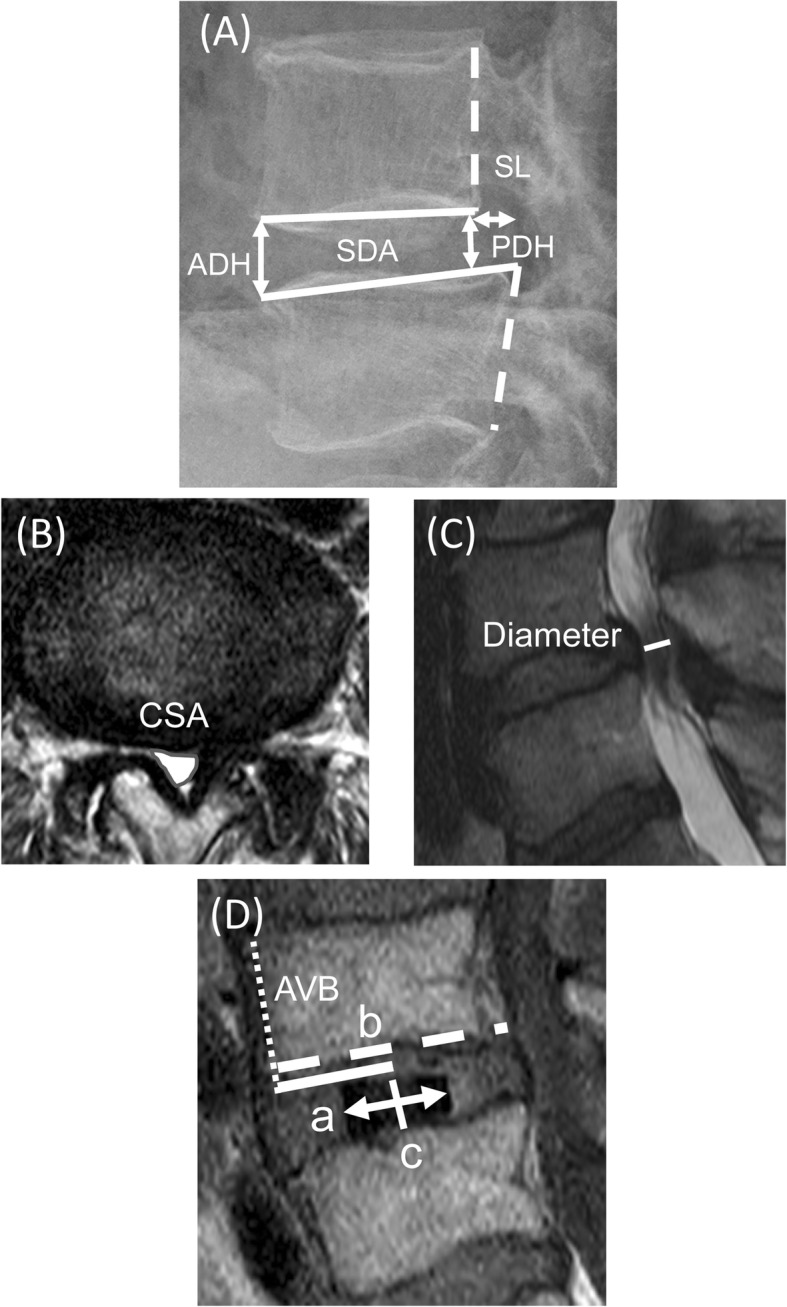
A radiographic analysis of XLIF cage positioning. (A) Plain lateral radiographs: segmental disc angle (SDA), disc height (DH), and slipping length (SL). (B) Magnetic resonance imaging (MRI)-T2 axial: cross-sectional spinal canal area (CSA). (C) MRI-T2 sagittal: diameter of the spinal canal. ADH; anterior disc height, PDH; posterior disc height. (D) The cage centre (c) is located by the midpoint between the anterior and posterior radiomarkers of the cage. The solid line (a) indicates distance between the anterior vertebral border (AVB) and the centre of the cage. The dotted line (b) indicates the anteroposterior width of the superior end plate. The cage positioning was measured using a Magnetic resonance imaging T1- weighted imaging and the value (a) divided by the full distance of the endplate (b) was expressed
